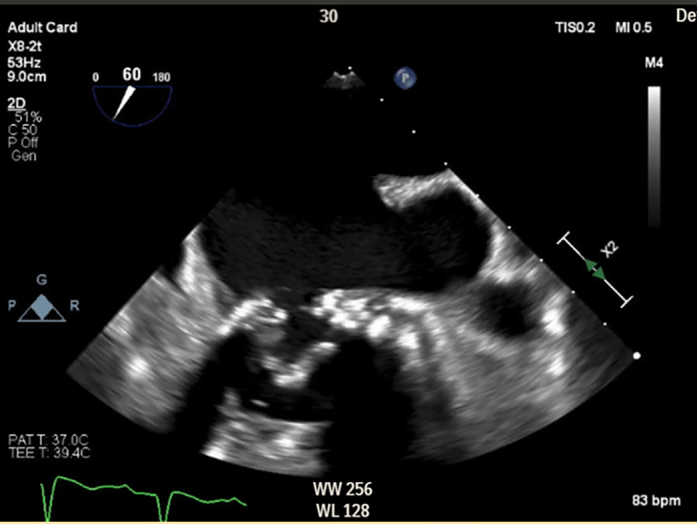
Transesophageal Echocardiography Showing Severe Mitral Calcification and Leaflet Immobility
